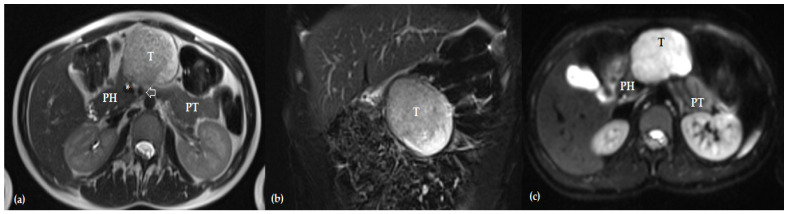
(a) Axial and (b) coronal T2-weighted magnetic resonance images showing a large, relatively exophytic, well-circumscribed mass in the pancreatic body (T) that is heterogeneously hyperintense relative to the nearby pancreatic parenchyma, compressing but without invading the portal vein (*) or superior mesenteric artery (white arrow); (c) axial diffusion-weighted magnetic resonance imaging showing the mass in the pancreatic body (T) with restricted diffusion compared with the nearby pancreatic parenchyma (PH—pancreatic head; PT—pancreatic tail).
